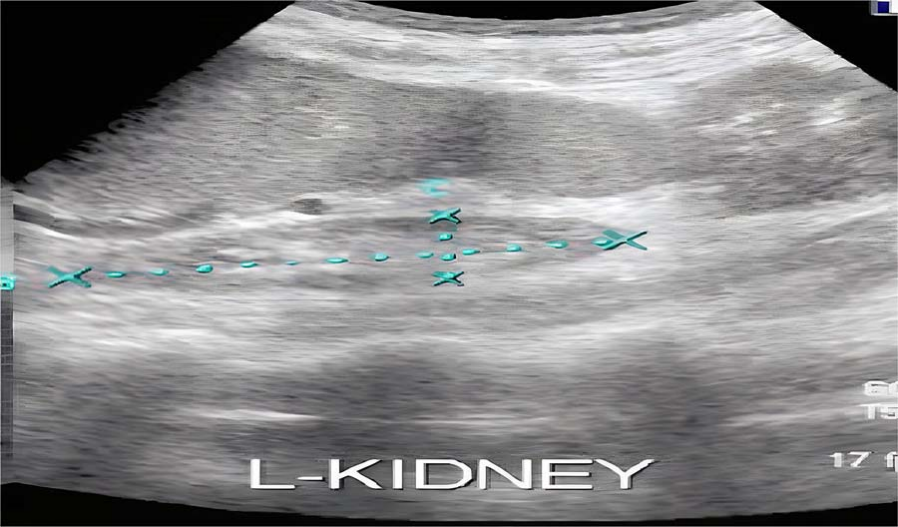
Ultrasound of the kidney showing an atrophic left kidney.
